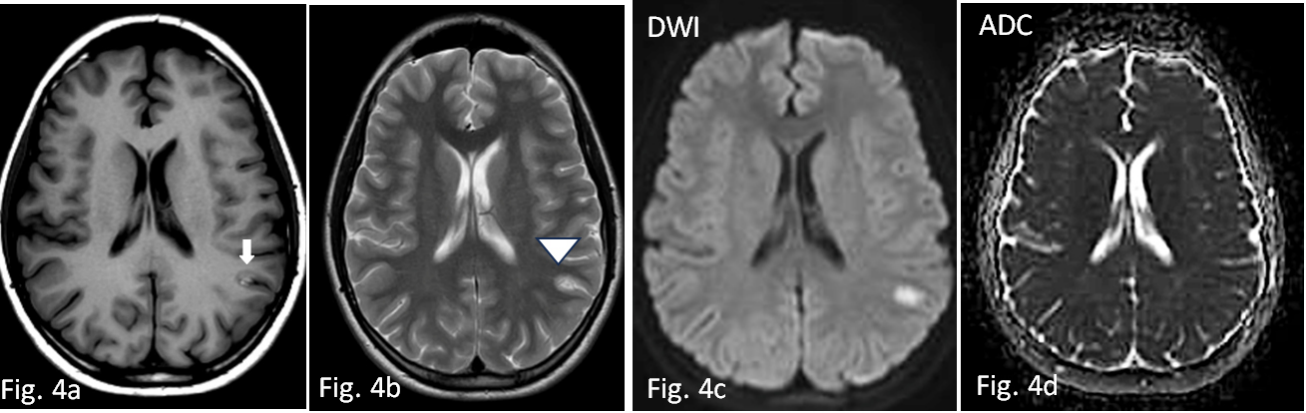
Control MRI examination performed twenty days later. Axial SE
